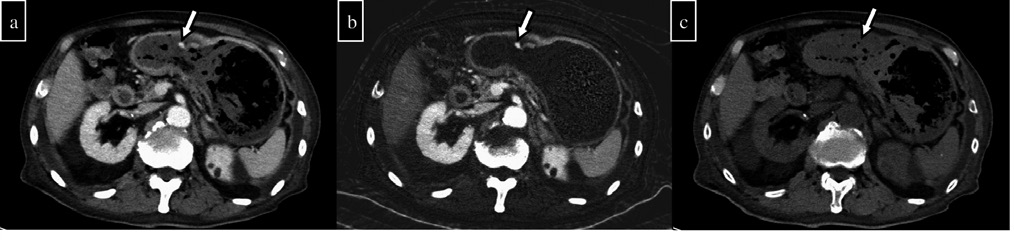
An 81-year-old man was hospitalised for heart failure because of atrial fibrillation. A single-phase contrast-enhanced CT was performed because of sudden haematochezia. (a) Conventional CT shows a high-density area in the stomach (arrow). (b) The iodine map shows high density in the same area and low density in all other gastric contents. (c) VNC shows a decrease in the density of the high-density area in the stomach on conventional CT. From (b) and (c), the presence of active bleeding in the stomach can be diagnosed. VNC, virtual non-contrast CT.
